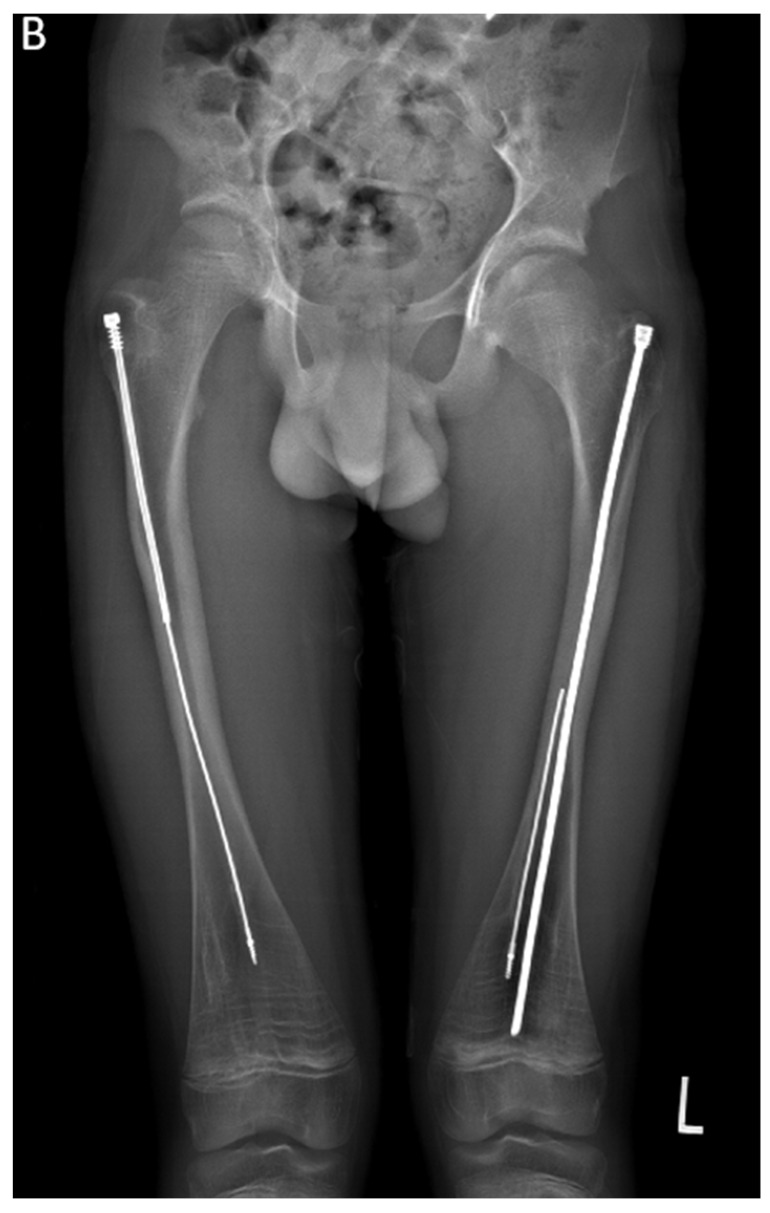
(A)—Pre-operative X-ray. (B)—Post-revision X-ray showing installed SLIM nail in the left femur.
